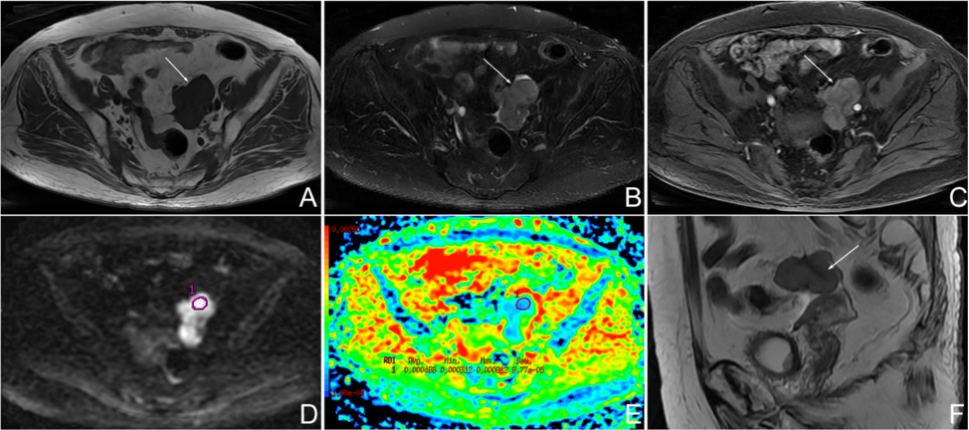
Female, 85 years, serous adenocarcinoma on the left ovary. a Axial T1WI showed a irregular mass with isointensity (*arrow*). b On axial fat-saturated T2WI, the mass was hyperintense (*arrow*) compared with the myometrium (not shown). c On axial contrast-enhanced fat-saturated T1WI, the mass (*arrow*) revealed less enhancement than myometrium (not show). d On DWI, the mass appeared as a very high signal (grade 3). e On the ADC map, the mean ADC value was 0.49 × 10^−3^ mm^2^/s. f On sagittal T2WI, the manifestation was similar to Fig. 6b
